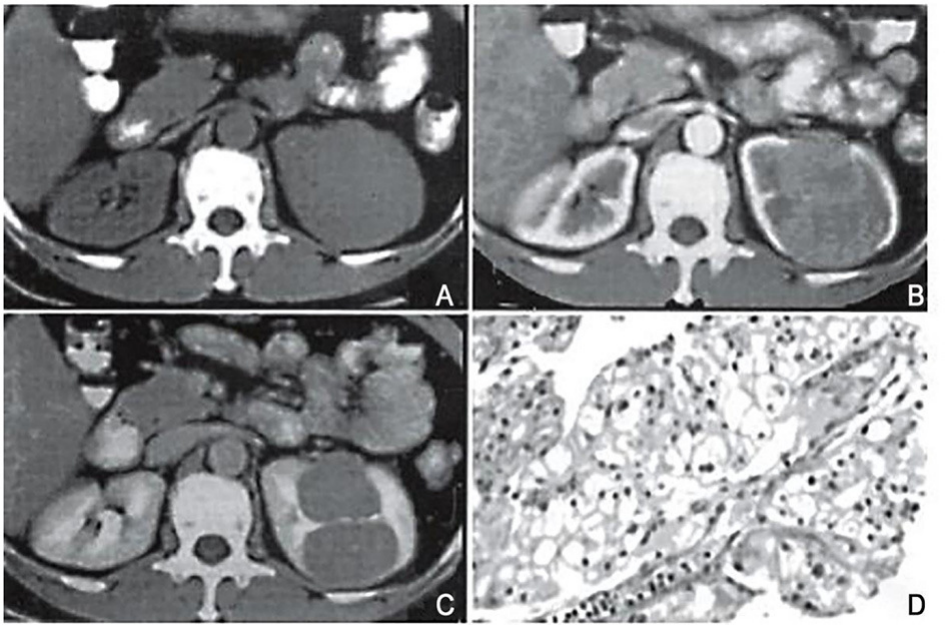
Case 1, female, 51 years old. CT classification: limited convex type. (A) Flat scan: an isobaric soft tissue mass in the upper part of the left kidney is partially protruding out of the contour of the kidney, with a smooth edge. The density of the lesion is uniform, and the boundary with the kidney tissue is unclear. (B) Arterial phase: the tumor shows obvious uniform enhancement, with clear borders and lobules. The lesion is locally convex but the surface is smooth. (C) Delayed scan: the tumor density is reduced, but the borders are clear, and a pseudo-capsule is formed around it. Surgery pathological biopsy (HEx200). The tumor tissue is arranged in a papillary shape. The image is a longitudinal section of a nipple, with a longitudinal section of a blood vessel visible in the center. Cancer cells are round-shaped, with transparent cytoplasm, and nuclei in the center or offset.
